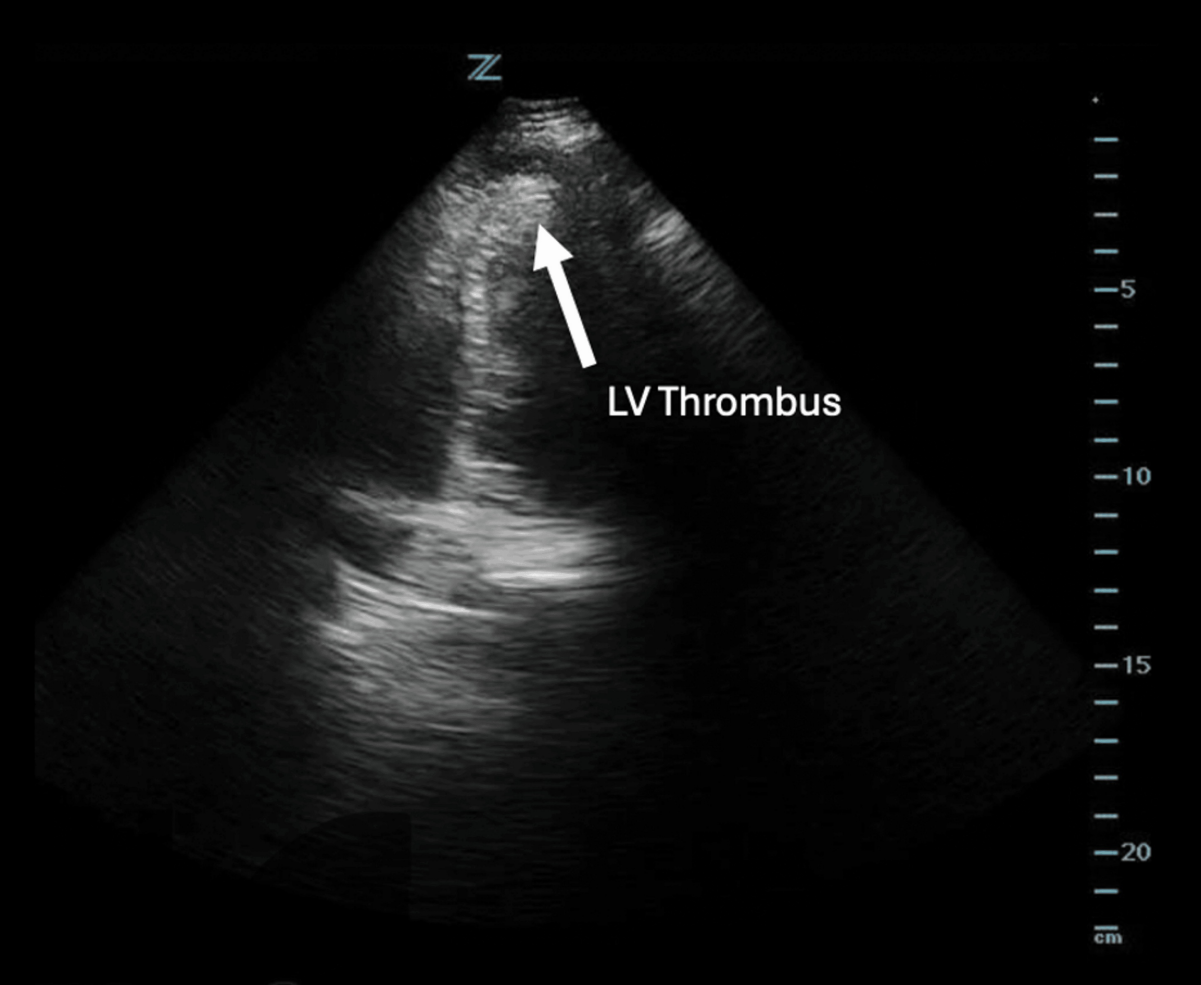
An apical four-chamber view of the left ventricle in Case 3, obtained using a phased array probe, demonstrating a small echogenic mass that is concerning for left ventricular thrombus.
